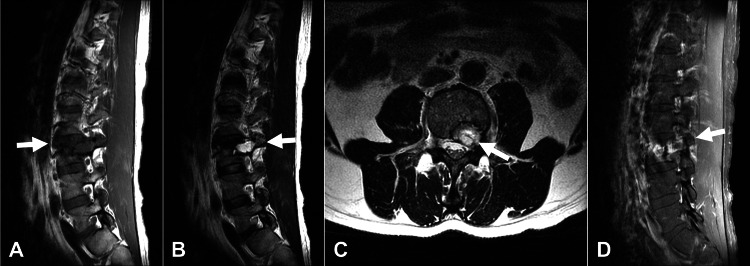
Magnetic resonance imaging of the lumbar spine. The anterior portion of the L3 vertebra with iso- and high-signal intensities on (A) T1-weighted image and (B) T2-weighted image, respectively (arrows). (B) Fluid-fluid level in the posterior portion of the vertebral body and left pedicle (arrow). (C) The lesion compresses to the left side of the dura and left L4 nerve root at the lateral recess (arrow). (D) The anterior portion of the vertebral body contrasted on gadolinium-enhanced MRI (arrows). MRI: magnetic resonance imaging
